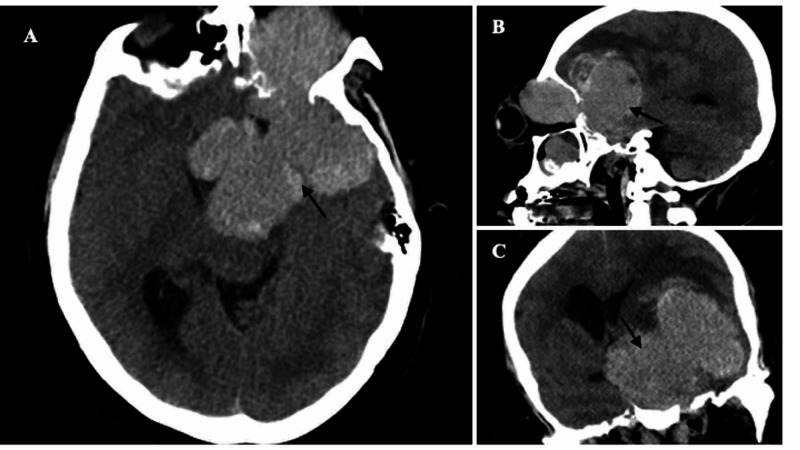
Non-contrast CT scan on presentation. (A) Axial, (B) sagittal, and (C) coronal views showing the 10 cm x 7 cm x 6 cm tumor invading the orbit, paranasal sinuses, and frontotemporal lobes and exerting mass effect on the brain parenchyma and brainstem.
